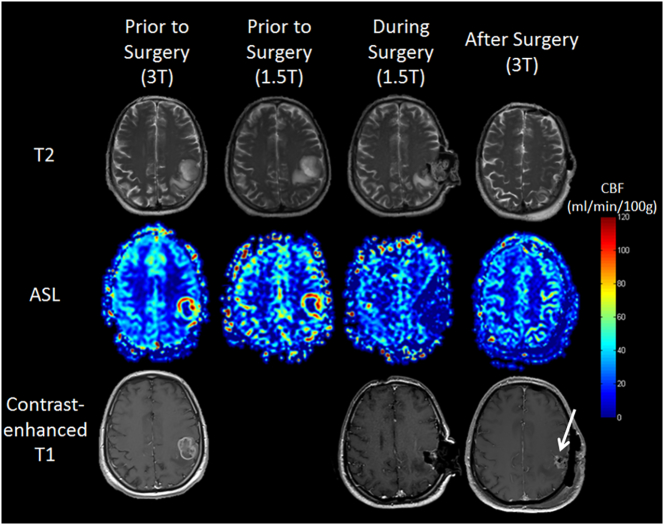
Example of a total resection (patient No. 1, Table 2). Images were acquired on the 3T and 1.5T scanners preoperatively (first and second left column), intraoperatively on the intraoperative scanner (third column), and postoperatively again on the 3T (right column). The contrast-enhanced T1-weighted images are shown in the bottom row. The ASL scans depicted the total resection in terms of no elevated CBF during and after surgery. Note that T1 weighted MR imaging “suggests” residual tumor tissue (arrow), which turned out to be an adjacent artery after including all postoperative imaging sequences.
